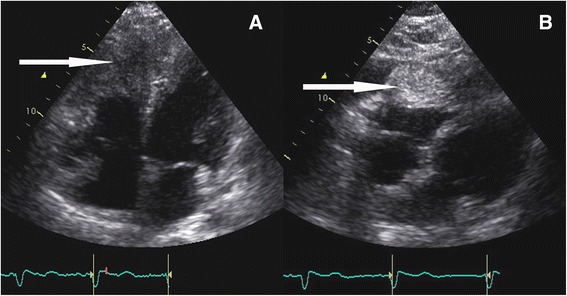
Apical four-chamber view (a) and subcostal four-chamber view (b) echocardiogram showing a large and homogenous mass in the right ventricle and pericardial effusion
